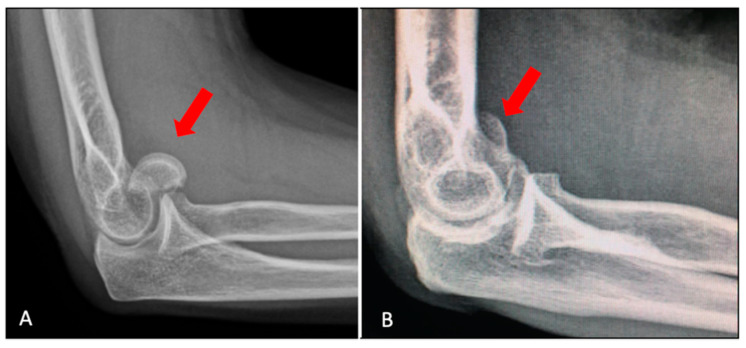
(A) Lateral X-ray showing the fracture fragment (red arrow) dislocated anterior to the distal humerus. (B) In cases of Bryan–Morrey Type II fracture, the injury looks like a loose body (red arrow).
